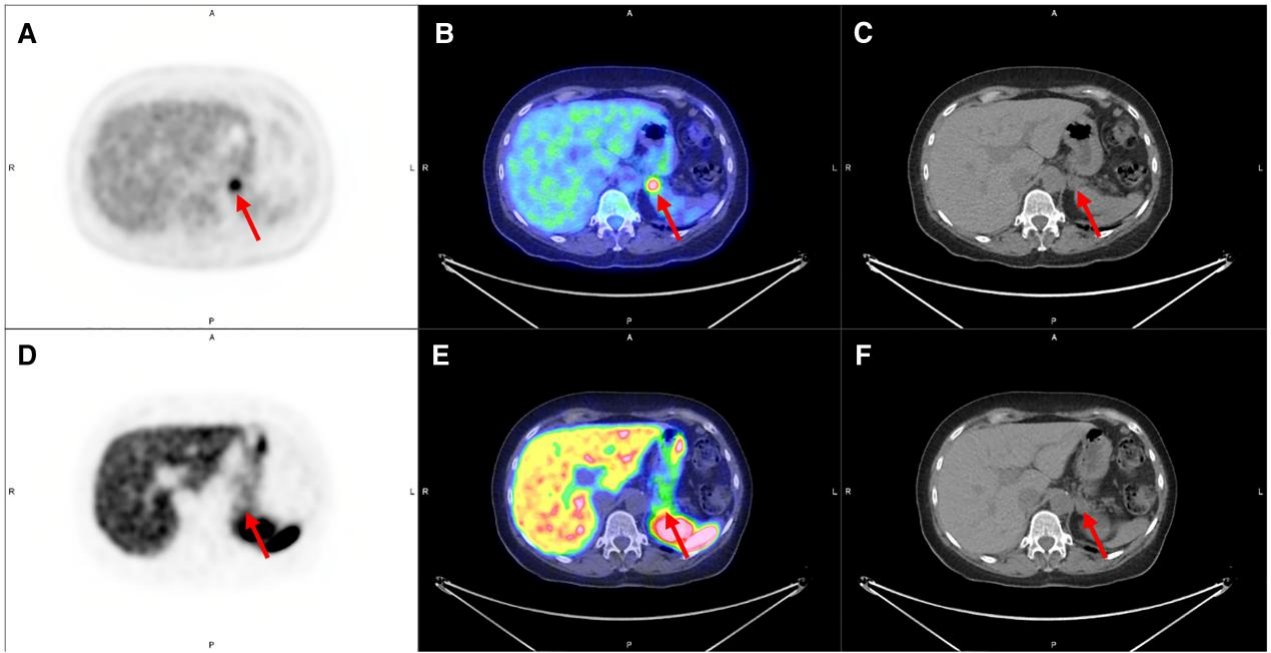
Select axial PET (A, D) CT (C, F) and PET/CT fusion (B, E) slices of a pancreatic tail lesion (arrow) with intense focal FDG uptake on [18F]FDG-PET/CT (A, B, C) and uncharacteristic absence of increased uptake on [68Ga]DOTATOC PET/CT (D, E, F) corresponding to a well-differentiated grade 1 pancreatic neuroendocrine tumor secreting ACTH.
